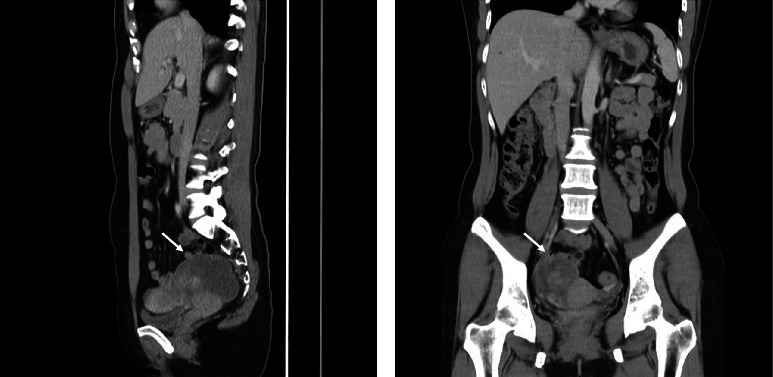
Abdominal computed tomography of the tumor. The tumor was with solid and cystic components in the right pelvic cavity. (a) Sagittal view (arrow), (b) coronal view (arrow).
